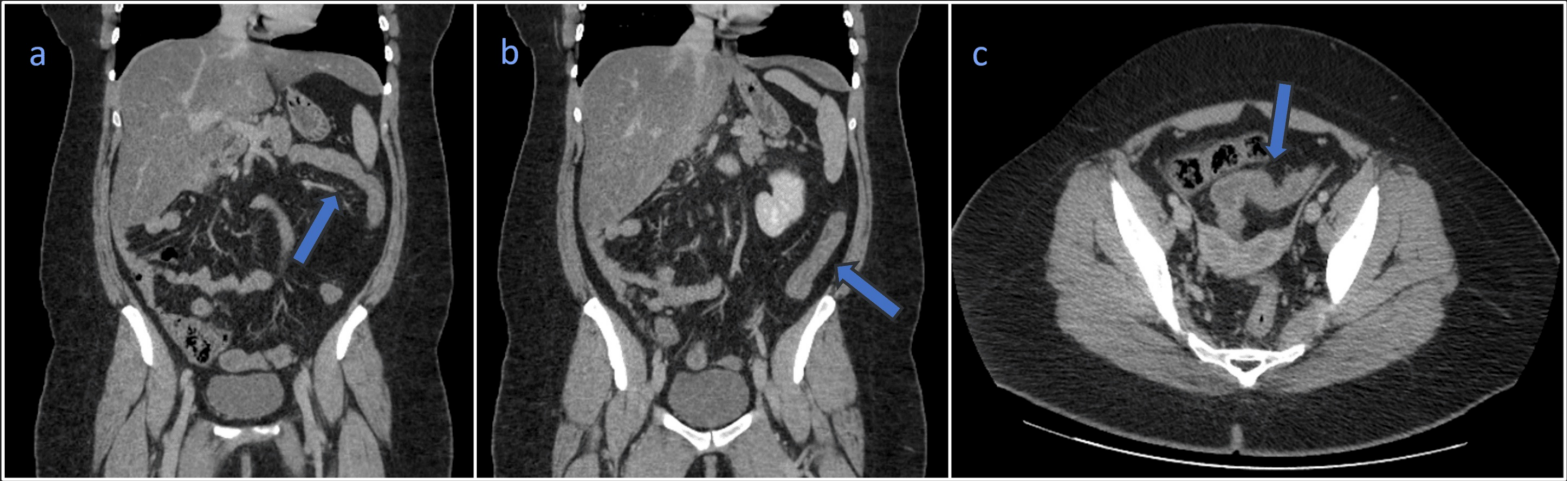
Abdominopelvic computed tomography scan (a) Coronal view showing signs of colitis in the splenic flexure; (b) Coronal view showing signs of colitis in the descending colon; (c) Axial view showing signs of colitis in the sigmoid colon.
